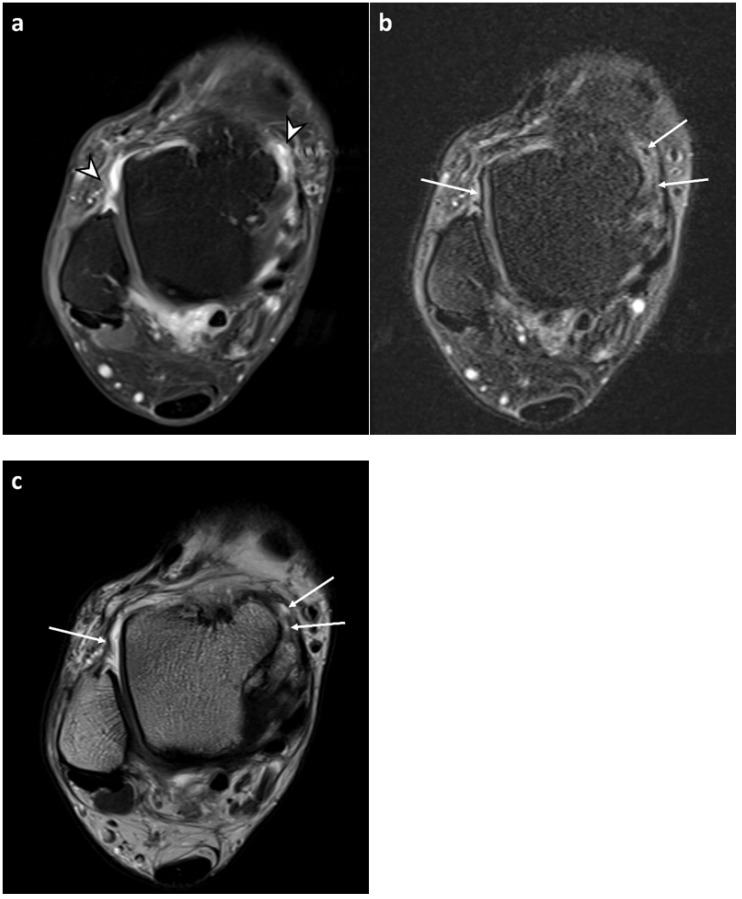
A 68-year-old male with osteoarthritis of the ankle joint. (a) Axial contrast-enhanced, T1-weighted sequence (CE-T1) with fat suppression image demonstrates enhanced synovium (arrowheads) with good tissue contrast. Both readers assigned a synovial visibility grade of 4 (fully visible synovium with good tissue contrast) in all joint compartments. For synovial thickness score, reader 1 assigned 2 (maximum thickness ≥ 4 mm), 1 (maximum thickness 2–4 mm), 1, and 2 and reader 2 assigned 2, 2, 2, and 2 in the anterior recess, anteromedial gutter, anterolateral gutter, and posterior recess, respectively. (b) Axial fluid-attenuated inversion recovery sequence with fat suppression (FLAIR-FS) image in the same level demonstrates dark signal intensity foci (arrows), suggesting suppressed joint fluid signals. Both readers assigned a synovial visibility grade of 4 in all joint compartments. For synovial thickness score, reader 1 assigned 2, 1, 0 (maximum thickness < 2 mm), and 1 and reader 2 assigned 1, 2, 0, and 1 in the anterior recess, anteromedial gutter, anterolateral gutter, and posterior recess, respectively. (c) Axial T2-weighted image in the same level shows joint fluid (arrows) at the location corresponding with dark signal intensity foci in FLAIR-FS image.
